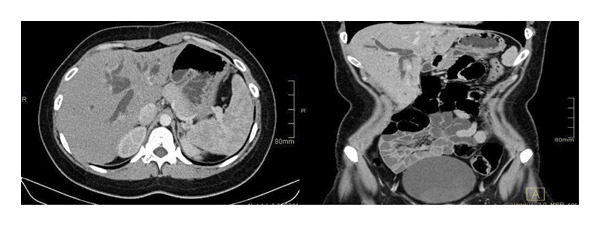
Axial venous phase CT: at the hepatic hilum (central bile duct level), an iso to hypodense, hypovascular mass lesion is seen, causing bile duct dilation, barely distinguishable from the surrounding parenchyma.
